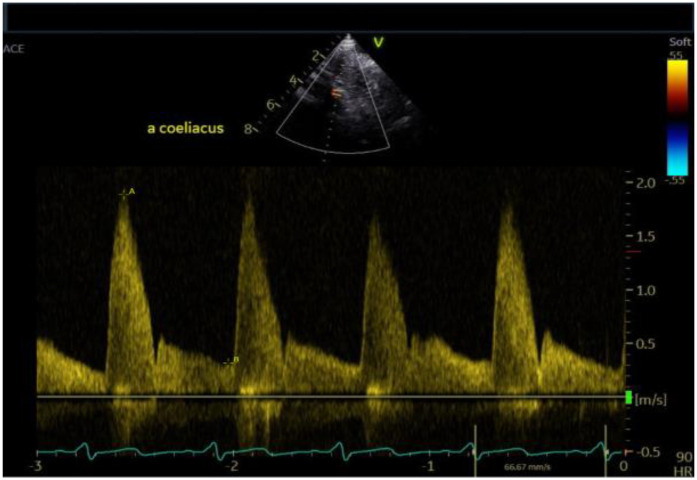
The blood flow of the mesenteric arteries are captured with e pulsed-wave Doppler from a sagittal subcostal view. Peak systolic velocity (A) and end-diastolic velocity (B) is measured, and resistance index is calculated.
